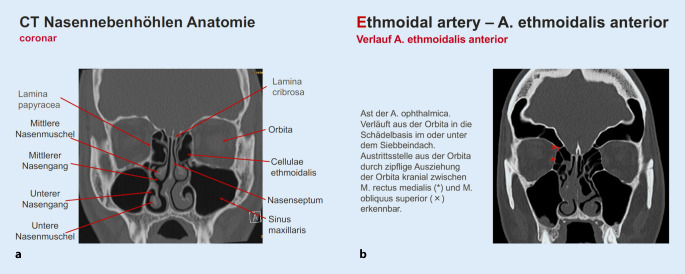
Der zweite Teil des E‑Learnings diente der Einführung der CLOSE-Kriterien. Die unterschiedlichen Strukturen wurden auf CT-Beispielbildern erläutert und deren klinische Relevanz ausgeführt.
